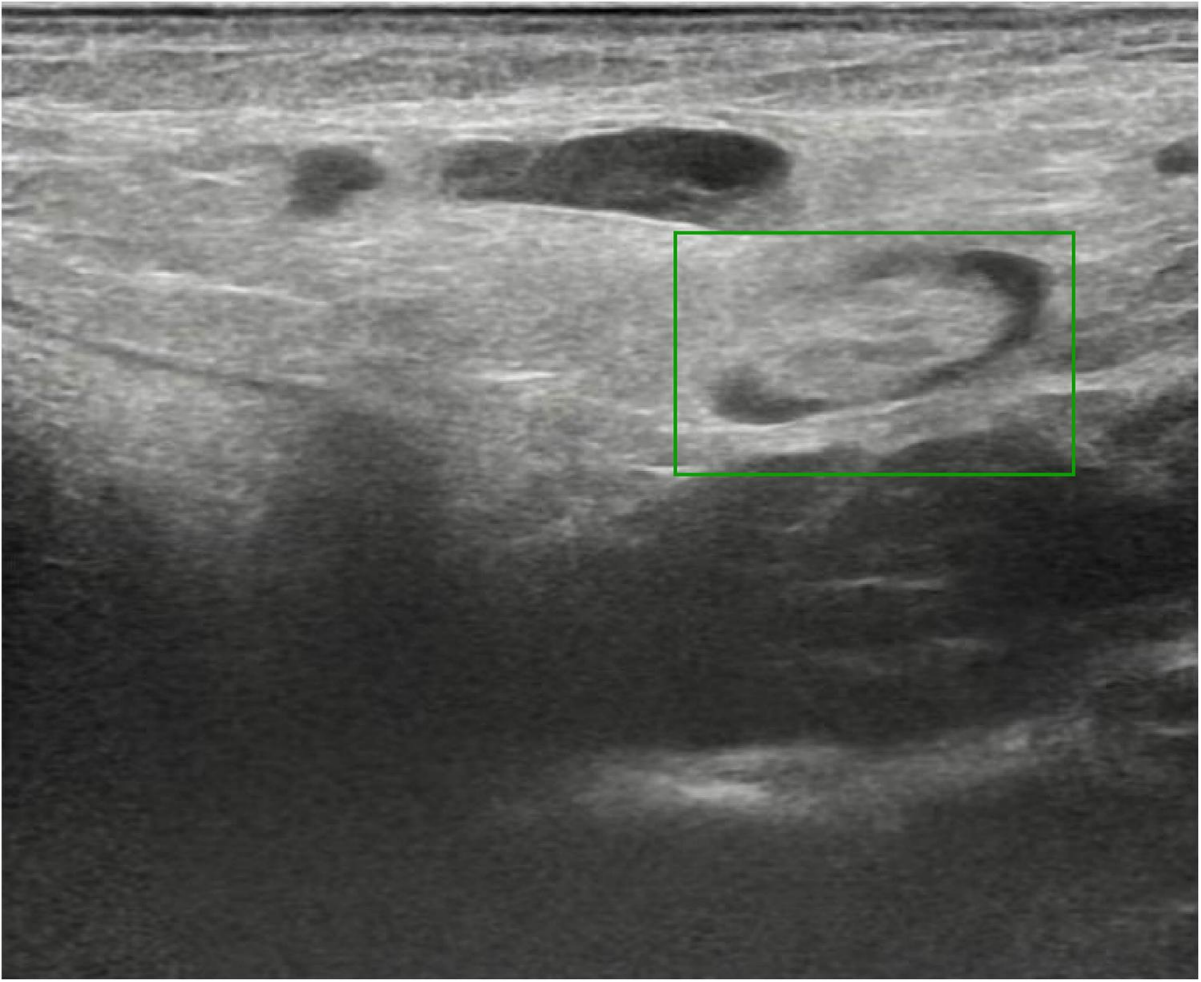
The post-operative B-ultrasound scan indicated that the left testicle was positioned within the scrotum (green box).
